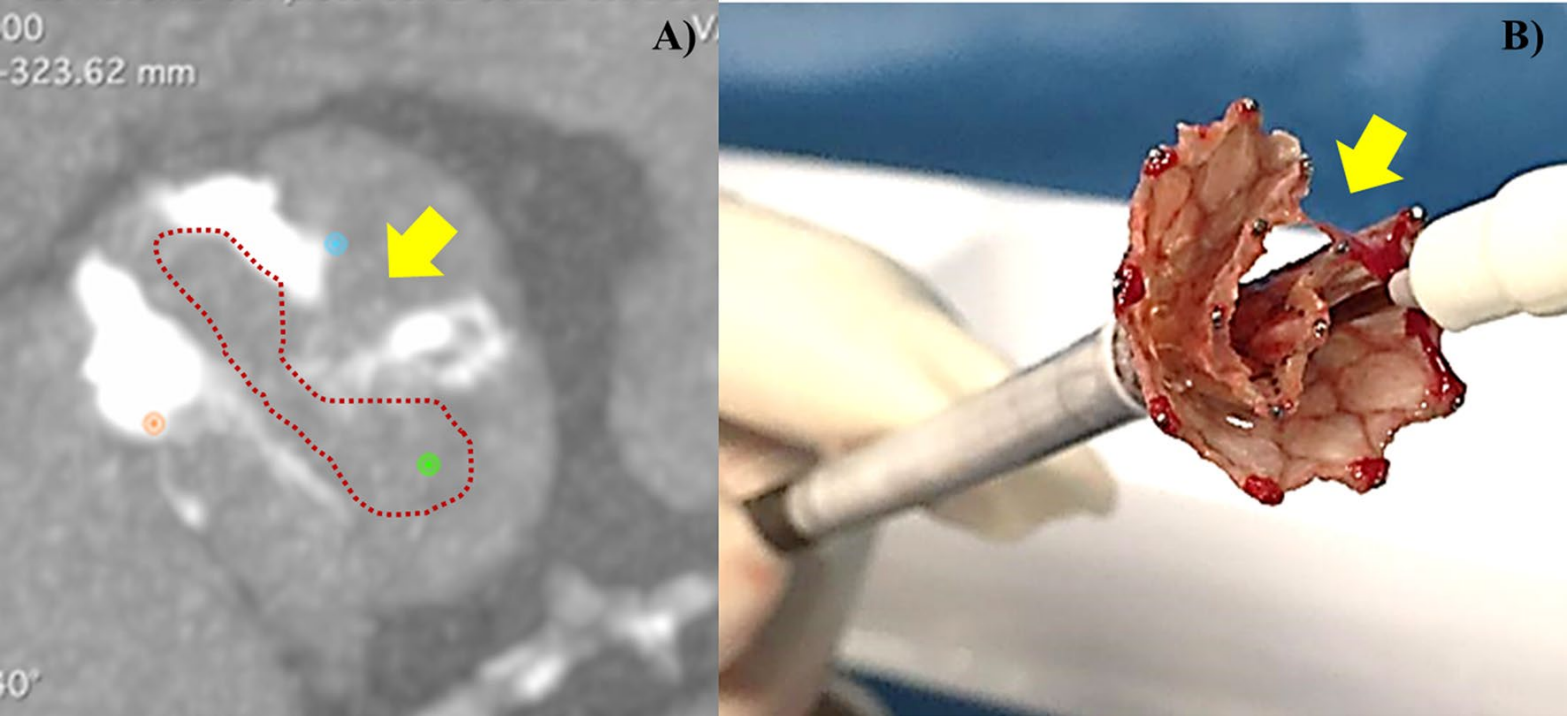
Panel A Pre-procedural images of computed tomography (CT) angiography with tridimensional reconstruction of aortic valve showed a bicuspid aortic valve (Type 1, L–R by Sievert Classification) with partially calcific raphe. The red dotted line reproduces the profile of the aortic valve orifice with evident protrusion of raphe (yellow arrow). Panel B Intraprocedural photographic image of infolded Evolut R THV with large invagination of the frame (yellow arrow) that exactly reproduces the aortic valve orifice profile as evidenced by CT (see Panel A)
